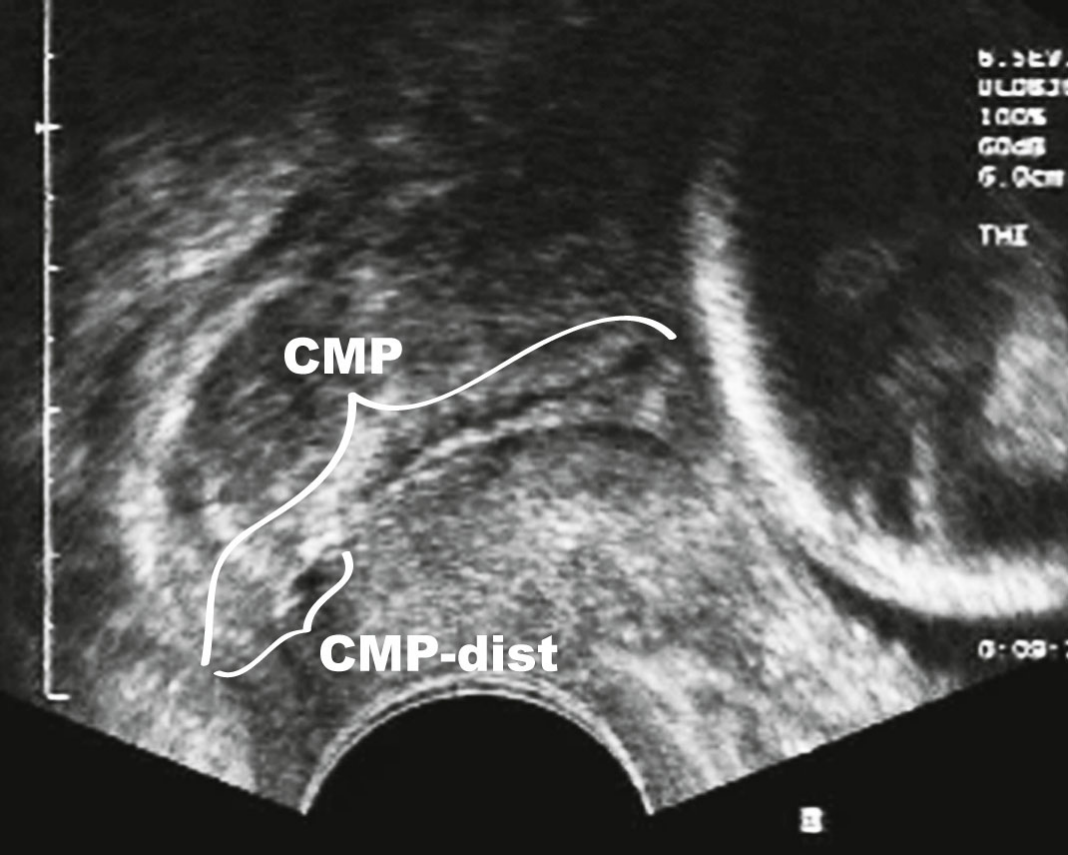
Definition of the CMP-dist. Ultrasonography showing the CMP in the cervical canal of a pregnant woman (gestational age 22 weeks). CMP-dist is defined as the part of the plug closest to the vaginal microflora.
